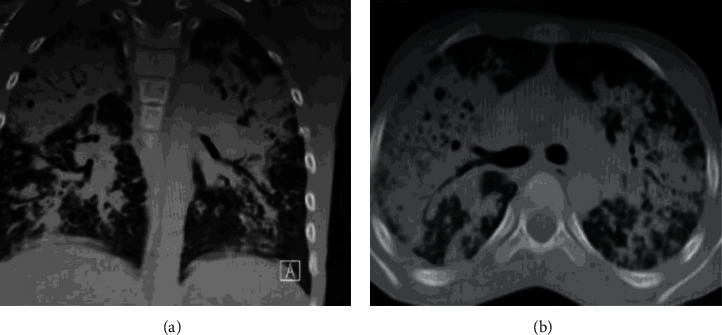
The chest CT scan showed patchy alveolar opacification (a) with multiple superior mediastinal lymph nodes (b).
